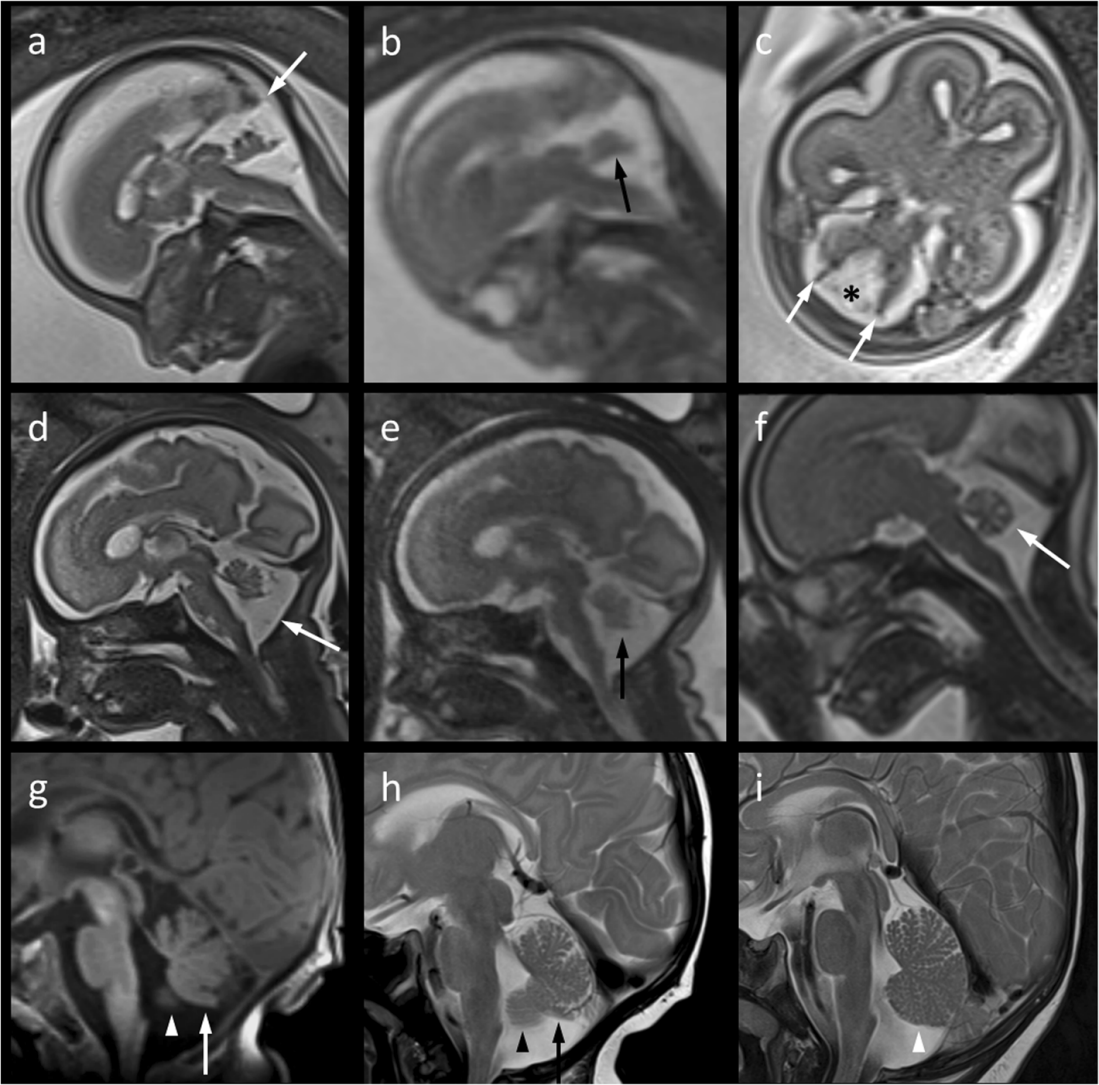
a–c Mid-sagittal (a, Half-Fourier Acquisition Single-Shot Turbo Spin-Echo [HASTE]; b, True Fast Imaging With Steady-State Free Precession) and axial (c, HASTE) T2-weighted 3 Tesla magnetic resonance (MR) images of the fetal brain at 21 weeks‘gestation suggesting a moderately hypoplastic cerebellar vermis with a flattened fastigial point (b, *arrow*) and moderately increased tegmento-vermian angle of about 35°, but normal torcular position (a, *arrow*). Lateral septa (c, *arrows*) in the posterior fossa are believed to belong to Blake’s pouch (c, *asterisk*). d, e Follow-up imaging on the same scanner at 31 weeks‘gestation shows nearly normal rotation of the vermis in a slightly enlarged posterior fossa (d, *arrow*) suggesting delayed fenestration of Blake’s pouch. Mild infero-posterior vermian hypoplasia (e, *arrow*) may be suspected from early third-gestational MR imaging (MRI) (d, e) This finding becomes more evident if compared to the sagittal T2 HASTE image of the cerebellar vermis (f, *arrow*) in a fetus at 24 weeks‘gestation scanned for suspected pulomonary sequestration on the same MR system. g, h A similar pattern is depicted by postnatal sagittal MRI (g, T1 Magnetization-Prepared Rapid Gradient-Echo; h, T2 Turbo spin echo) at the age of 12 weeks. Size and shape of the cerebellar vermis imply that it is mildly hypoplastic and its posterior lobe has experienced mass effect due to prolonged persistence of Blake’s pouch. Further, partial volume of cerebellar hemisphere (g, h; *arrow heads*) adjacent to the foramen of Magendie has to be considered. i For comparison of cerebellar volume and fissuration, the sagittal T1-weighted MR image in a 4-month-old, clinically inapparent infant scanned for a temporopolar arachnoid cyst – known from pre- and postnatal ultrasound – is given here
